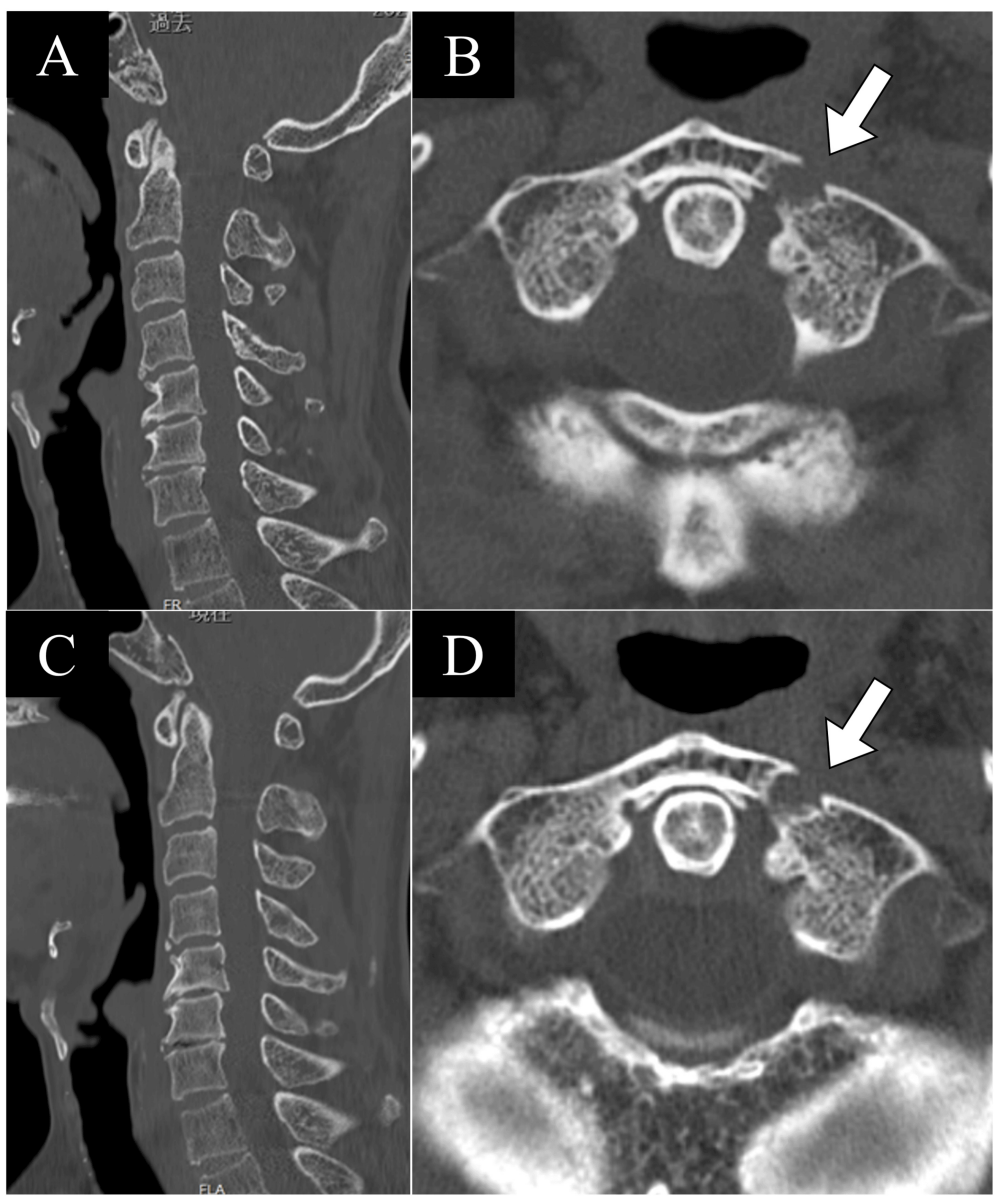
Cervical spine CT at four months and one year post-injury. A, B: Cervical spine CT at four months post-injury. A: No displacement was observed in the occipital bone or C4 vertebra. B: Callus formation was confirmed at the fracture site of the atlas (C1). C, D: Cervical spine CT at a year post-injury. C: No displacement of the C4 vertebra was observed, and bone healing was achieved in the occipital bone. D: Bony bridging was confirmed at the fracture site of the atlas (C1), indicating partial bone healing.
